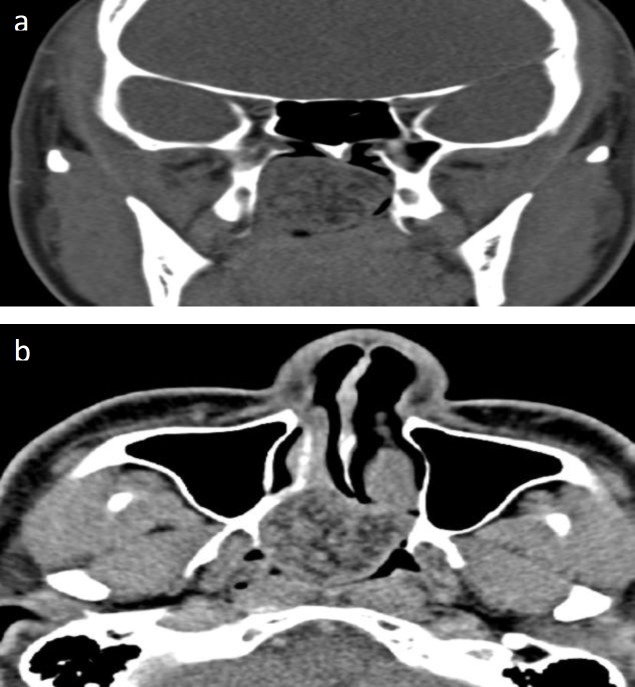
a) Axial and b) coronal view a CT scan of the paranasal sinuses showing a lobulated mass arising from the posterior end of the right inferior turbinate extending to the nasopharynx
